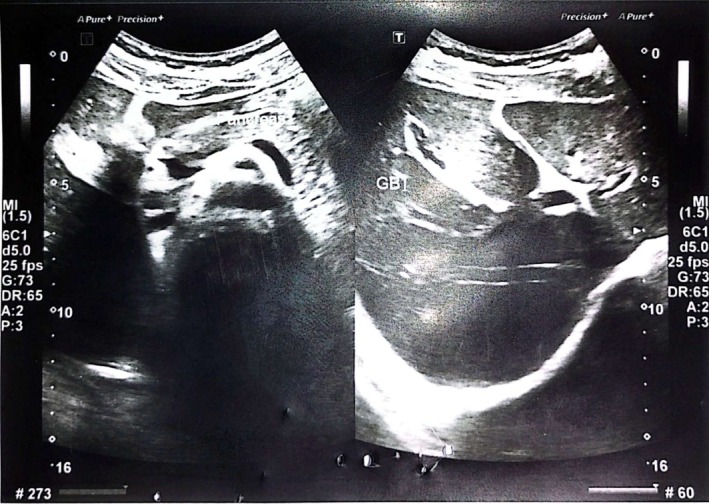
Close‐up ultrasound view of the periportal regions demonstrating the starry sky pattern. Bright portal vein walls contrast with the darkened hepatic parenchyma due to inflammation and fluid accumulation.
